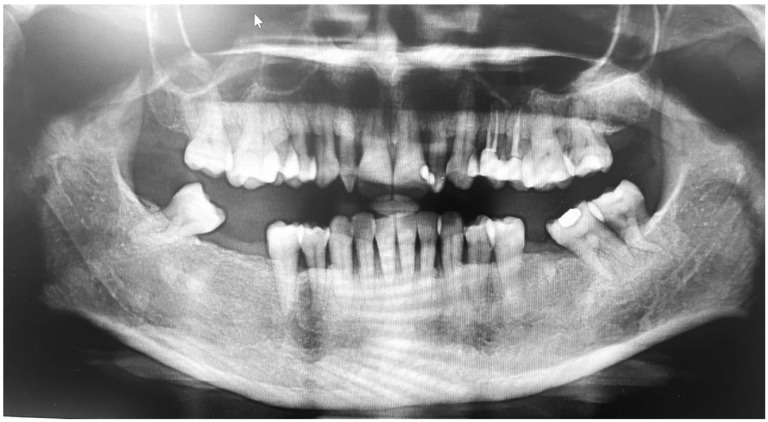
Orthopantomogram showing generalized horizontal bone loss, multiple missing teeth, and restorations.
